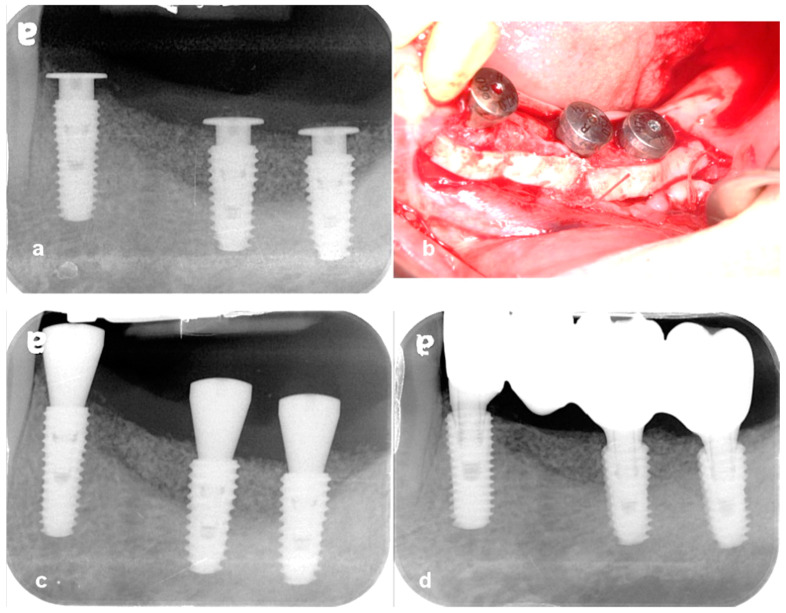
(a) A postoperative periapical radiograph reveals bone grafting on the defect. (b) The uncovering was performed after 22 weeks of healing. A suture-less free gingival graft was utilized to obtain attached gingiva. (c) A periapical radiograph reveals stable ridge augmentation over implant platform. (d) A periapical radiograph after the delivery of a final restoration.
